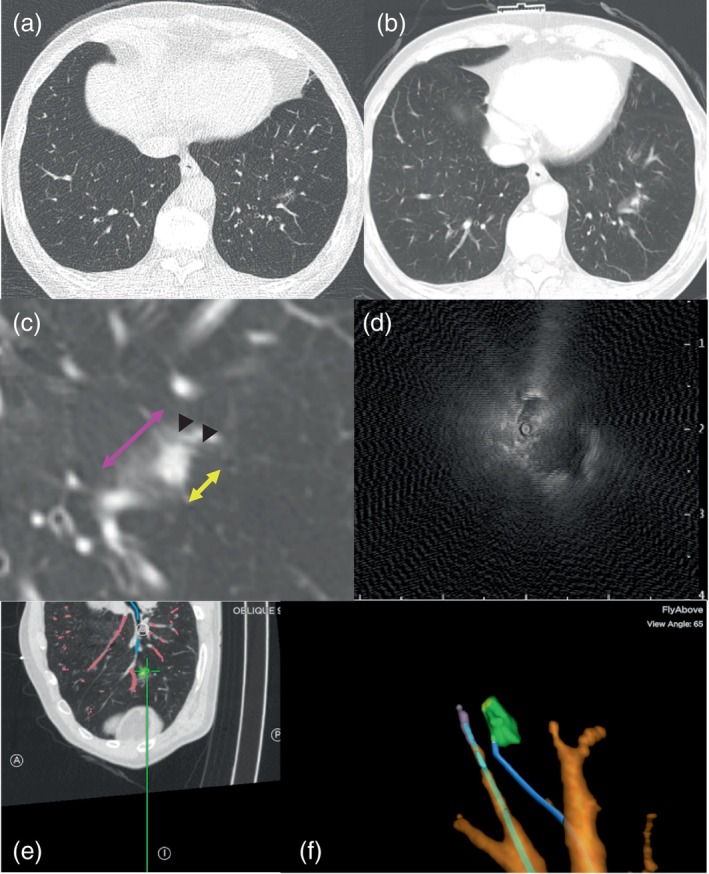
Example of a part‐solid nodule with a solid component smaller than 20 mm and bronchus type III. Compared with the CT findings from 1 year prior (a), the nodule in the left lower lobe had increased in size (b) and malignancy was highly suspected. However, the lung function of the patient was poor, making surgical resection infeasible, therefore a combination of ENB and RP‐EBUS was performed for a biopsy of the lesion. (c) The bronchus transversing around the nodule (arrowheads), a 9.6‐mm solid component (yellow arrow), and a 21.4‐mm whole lesion (pink arrow). (d) The eccentric nodule on RP‐EBUS. (e) The ENB system showing the nodule as the scope approaches. (f) The scope reaching the nodule. The patient was diagnosed with adenocarcinoma and received stereotactic body radiotherapy.
